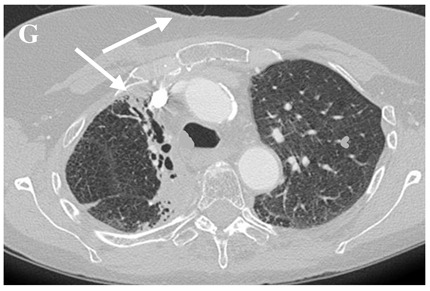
Axial CT chest (image G) showing dense fibrosis and bronchiectasis in the right upper lobe (RUL) post radiotherapy denoted by an arrow.
